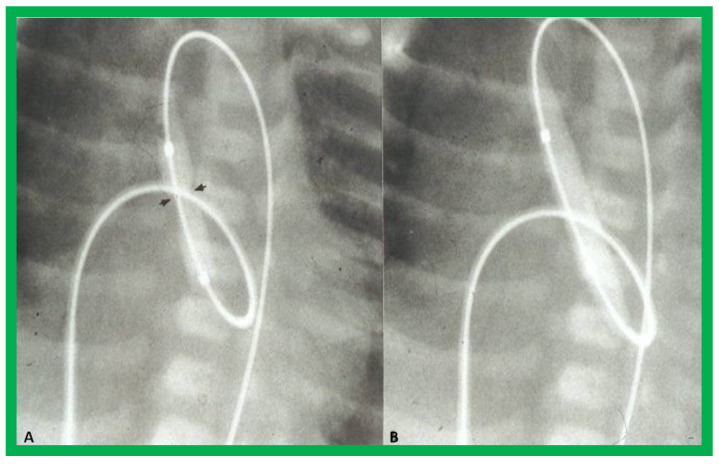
Selected cine-radiographic frames demonstrating the position of the balloon across the aortic valve, introduced anterogradely. Note the waisting (arrows) of the balloon during the initial phases of balloon inflation (A), which was completely abolished after full inflation of the balloon (B). Reproduced from Reference [54].
